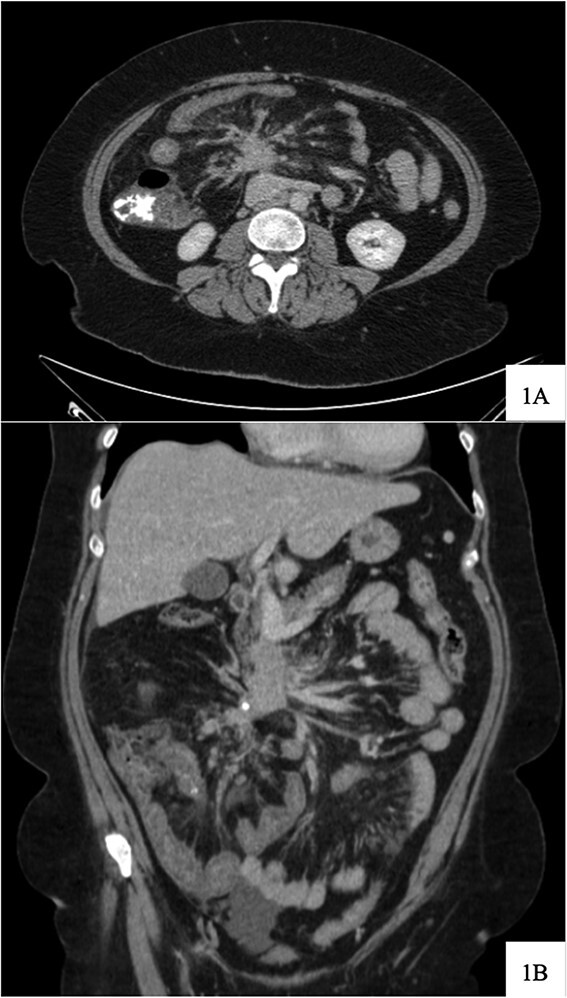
Computed tomography scan at time of diagnosis in September 2017 revealed a mass near the third portion of the duodenum and the root of the mesentery in the axial (A) and coronal (B) views.
